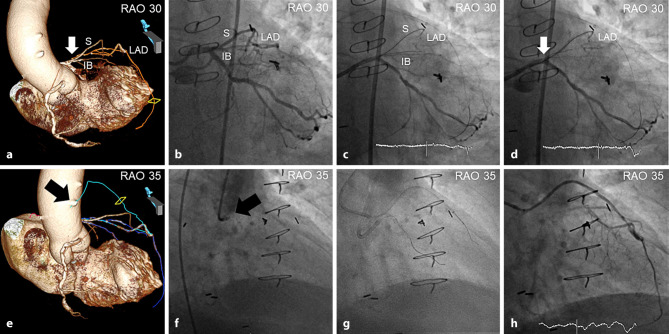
a–h Coronary computed tomography angiography (*CTA*) for clarification of proximal cap ambiguity and guiding recanalisation of the occluded venous graft for retrograde access to a chronic total occlusion. Three-dimensional CTA volume-rendered reconstruction showing the exact location of the proximal cap of a chronically occluded left anterior descending coronary artery (*LAD*) arising proximal to and above the septal (*S*) branch in the right anterior oblique (*RAO*) view (*arrow*). The exact course of the ostial LAD occlusion traversing between the septal and intermediate branch (*IB*) is displayed (a). Invasive coronary angiography failing to show the origin of the chronically occluded LAD (b). Advancement of the PILOT 200 guidewire (Abbott Vascular, Santa Clara, CA, USA) into the false lumen along the IB (c). Based on CTA images, the PILOT 200 guidewire was retracted and redirected pointing upwards and proximal to the septal branch, tracking the course of the LAD between the septal and IB (d). Three-dimensional CTA volume-rendered reconstruction depicting the exact location of the short stump (*black arrow*) as well as the course of the occluded venous graft to the chronically occluded LAD (e). Invasive angiography showing the stump (*black arrow*) of the occluded graft to the LAD (f). Based on CTA reconstructions aligned with the angulation of the C‑arm, the occluded venous graft was successfully recanalised using the PILOT 200 guidewire supported by a Caravel (Asahi Intecc, Nagoya, Japan) microcatheter (g, h)
